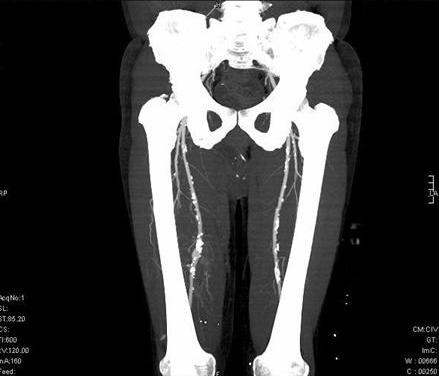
CT scan. Atheroma plaques in all arterial vascular beds focused (inferior limbs).
